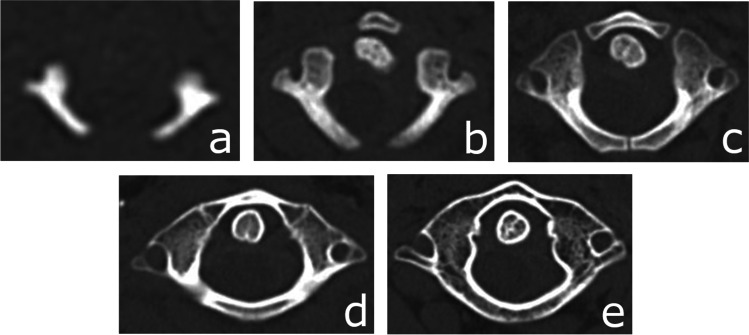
Computed tomography images depicting consecutive stages of ossification in the anterior arch of the atlas: A Stage 1 – no primary ossification center in the anterior arch of the atlas; B Stage 2 – the ossification center of the anterior arch of the atlas is present, its shape is irregular; C Stage 3 – a mature form of the anterior arch of the atlas, with open synchondroses; D Stage 4 – the anterior synchondroses are partially closed; E Stage 5 – complete closure of the anterior synchondroses
